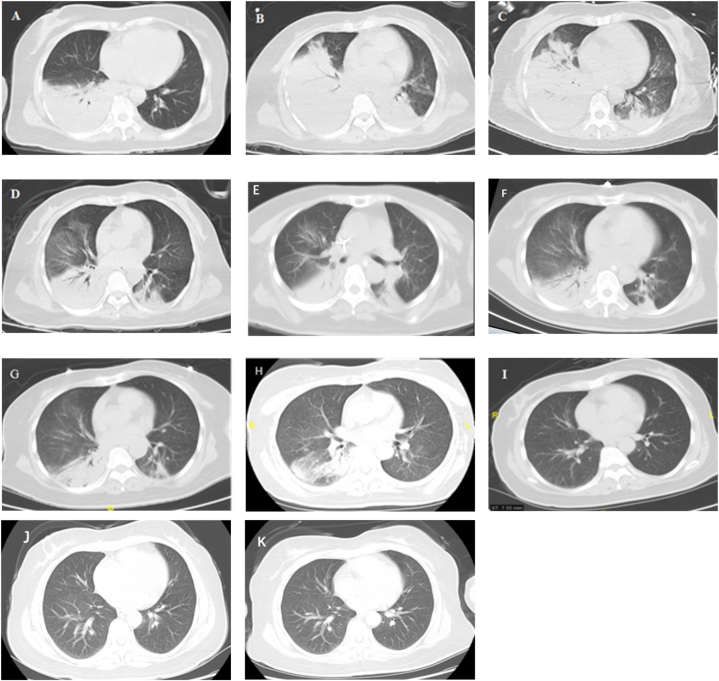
Lung computed tomography of the patient during hospitalization and follow-up. (A) On admission; (B) On the3^rd^ day after admission; (C) On the 5th day after admission; (D) On the 14th day after admission; (E) On the 17th day after admission; (F) On the 20th day after admission; (G) On the 25th day after admission; (H) On the 11th day of follow-up after discharge; (I) On the 74th day of follow-up after discharge; (J) On the 313th day of follow-up after discharge; (K) On the 502nd day of follow-up after discharge.
